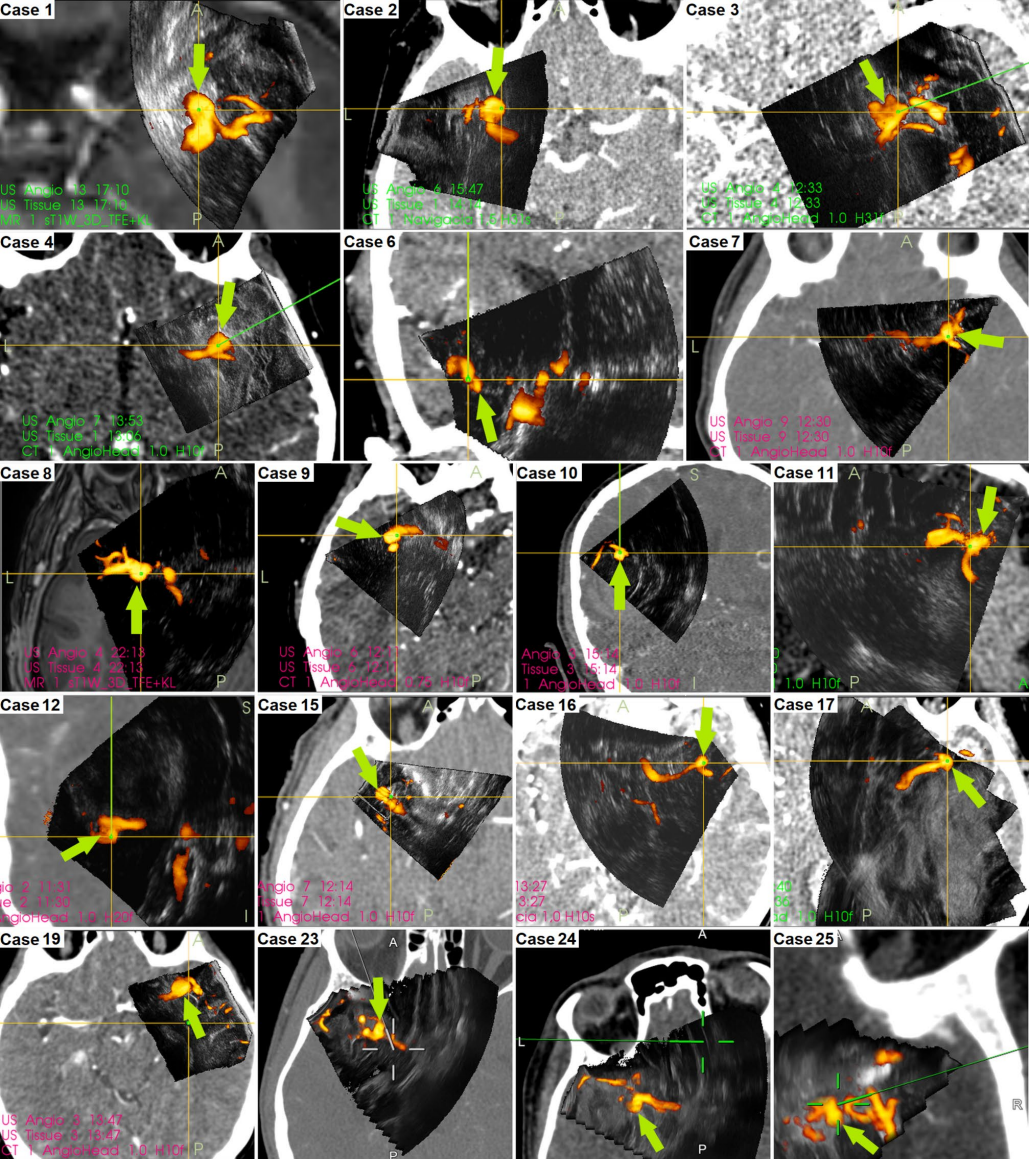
Overview of intraoperative 3D-US angiography images of 18 patients with distinctly depicted MCA aneurysms. Green arrows are pointing at the aneurysms. *In patient No. 14 no screenshots were available for technical reasons; however, the distinct aneurysm sonographic visibility could be reliably assessed based on the operating report
